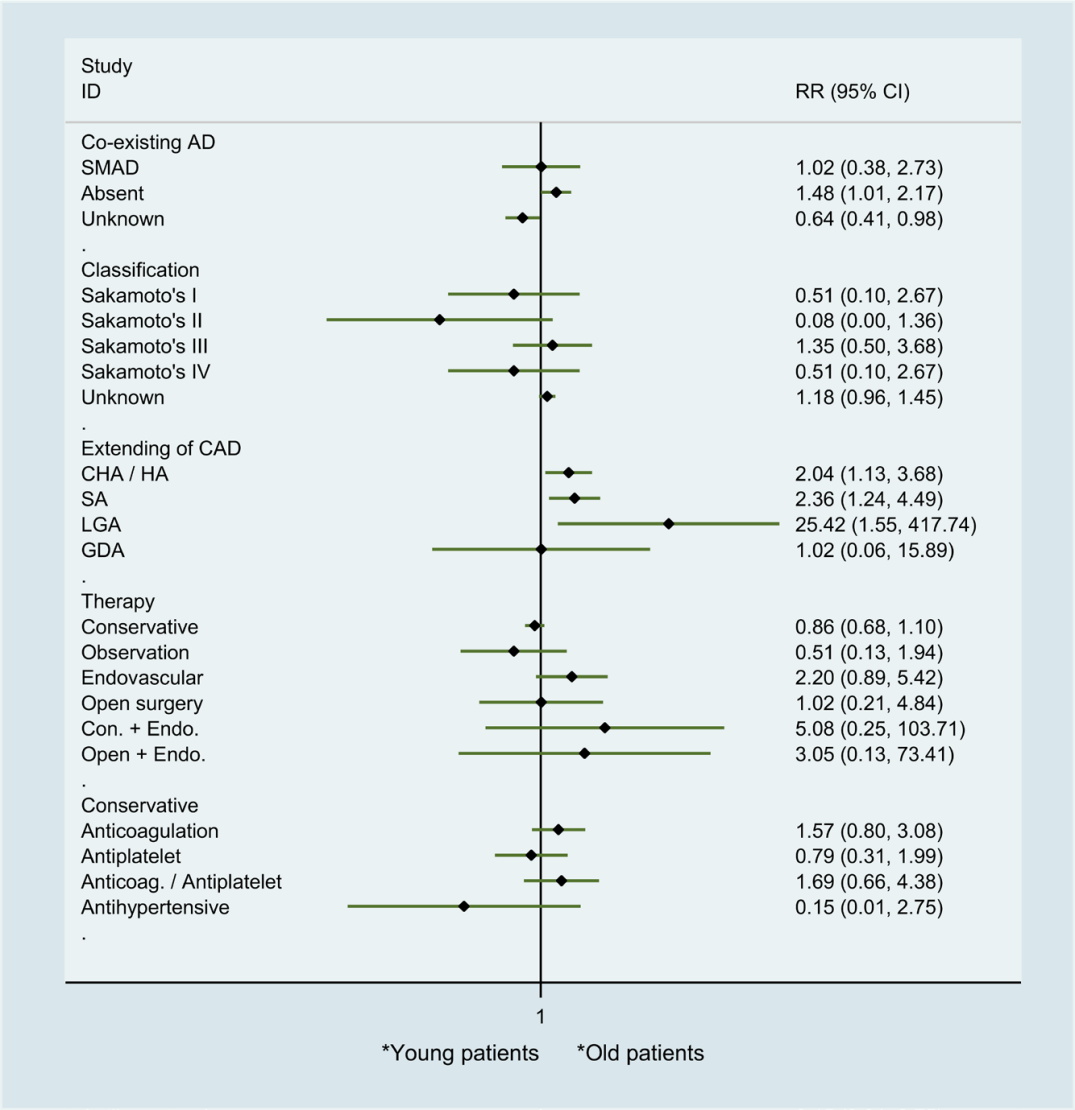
Comparison between young and old spontaneous ICAD patients regarding to radiographic characteristics and treatment. AD = artery dissection; CAD = celiac artery dissection; CHA = common hepatic artery; GDA = gastroduodenal artery; HA = hepatic artery; ICAD = isolated celiac artery dissection; ISMAD = isolated superior mesenteric artery dissection; LGA = left gastric artery; SA = splenic artery; endo. indicates endovascular; con. indicates conservative.
